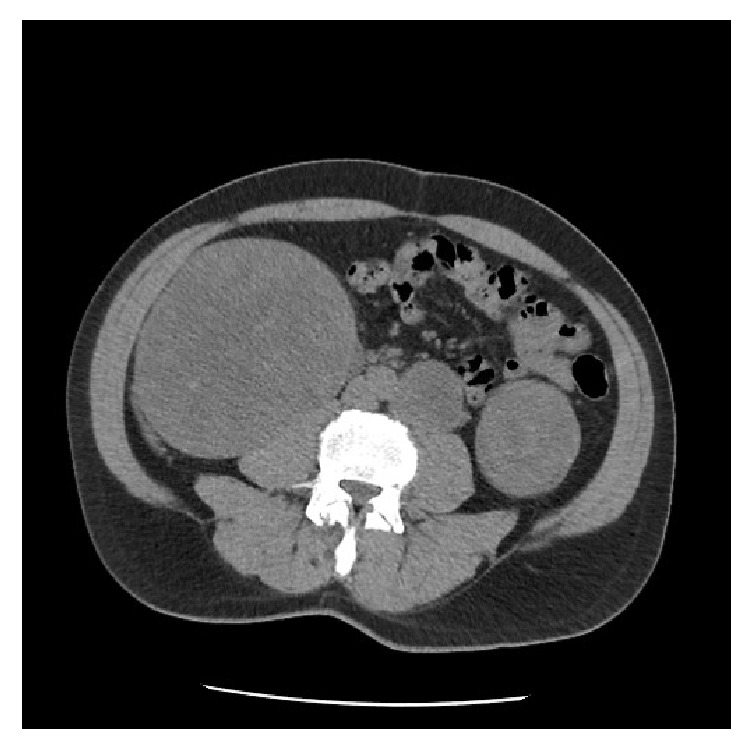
Computed tomography without contrast demonstrating left paraaortic mass, left interpolar renal mass, and large right renal mass.
